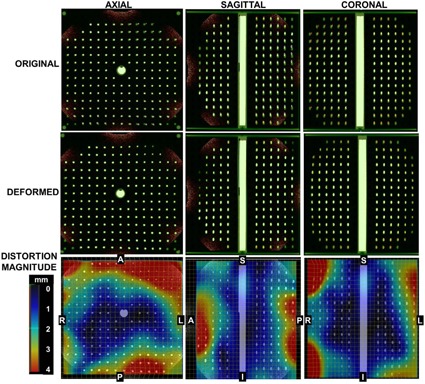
(Top row) Blended overlay of the original CT‐SIM (green) and MR‐SIM data (orange) prior to deformable image registration. Mismatches in marker location can be observed between datasets, particularly near the edges of the field of view. (Middle row) Overlay of CT‐SIM and MR‐SIM data after deformable image registration, showing the resolution of image distortions. (Bottom row) Vector distortion magnitude between the CT‐SIM and MR‐SIM datasets demonstrate the distortions occurring near the edges of the field of view. For reference, centroid‐to‐centroid marker spacing is 2.5 cm in‐plane and 2.7 cm along the z‐axis, and the registration field of view was $\sim 40\times 34\times 40\;{\text{cm}}^{3}$ in the right–left, anterior–posterior, and superior–inferior, respectively.
